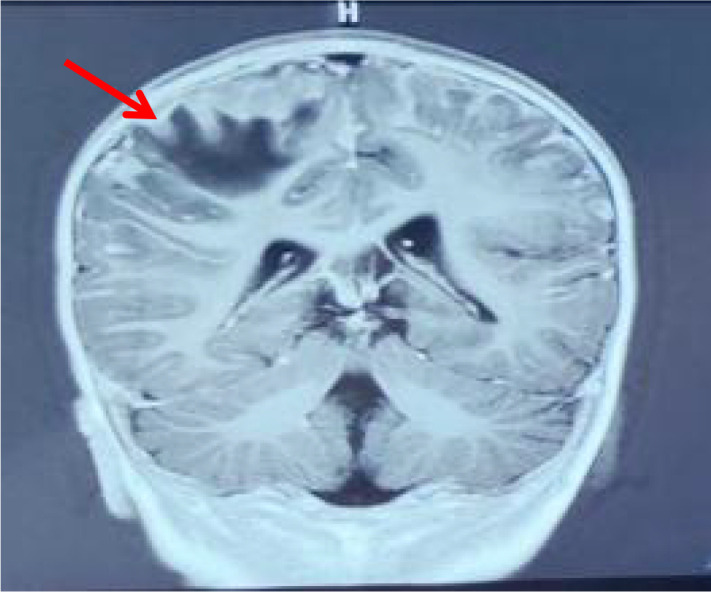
Cranial MRI revealed bilateral parietal parenchymal swelling and hypointense white matter lesions in the right parietal and frontal lobes.
